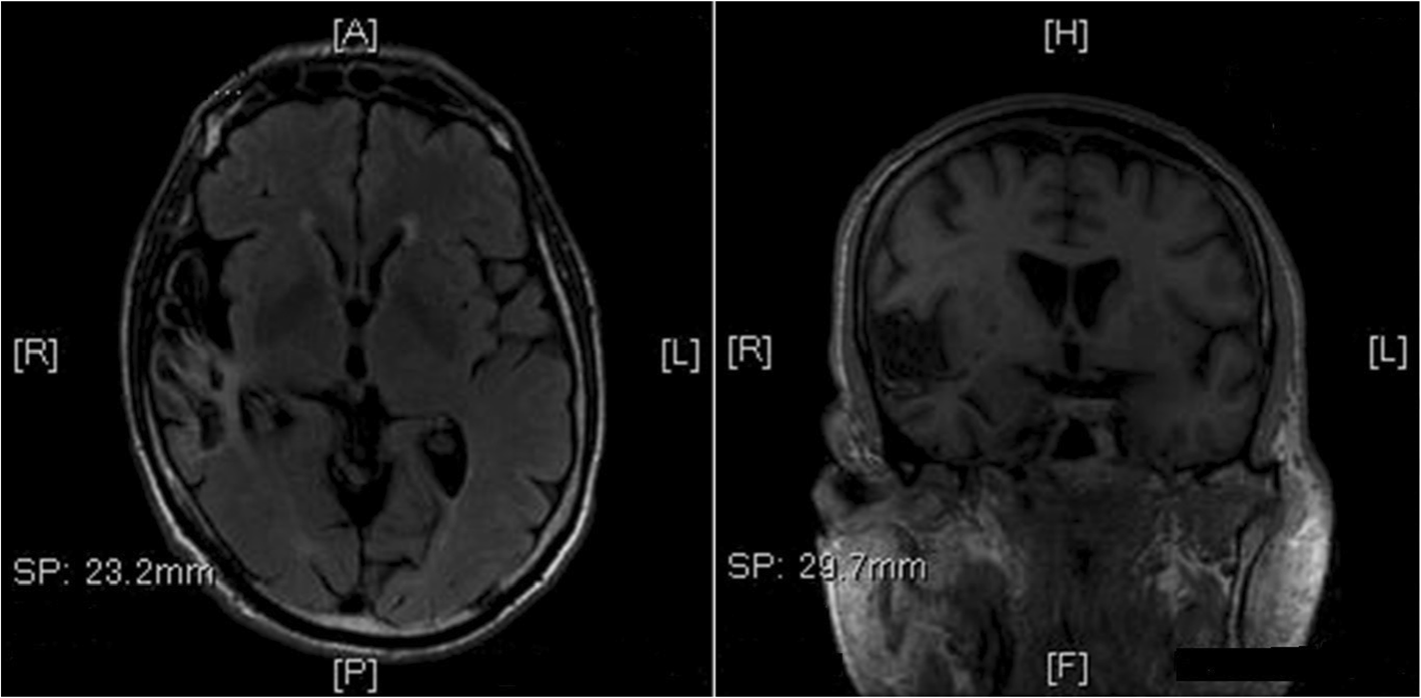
Axial FLAIR (left side) and coronal T1 3DTOF (right side) MRI images showing past right ischemic cortical-subcortical temporal-insular stroke
